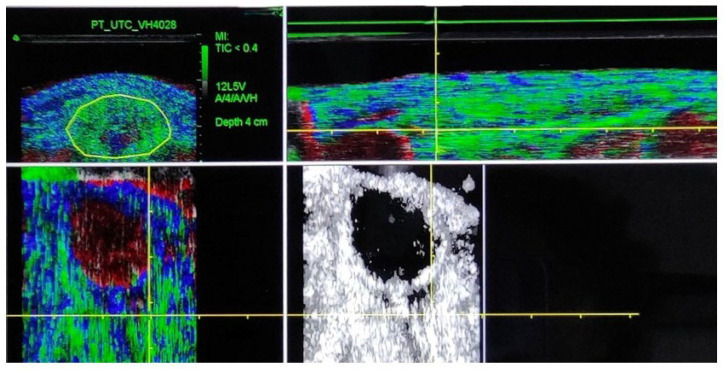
Ultrasound tissue characterization of the patellar tendon. Echo type classification; green = echo type I, blue= echo type II, red = echo type III and black = echo type IV: Right upper imagen (area of interest) = transversal view; left upper image = longitudinal view; right lower image = axial view; left lower image = axial view without UTC software classification.
